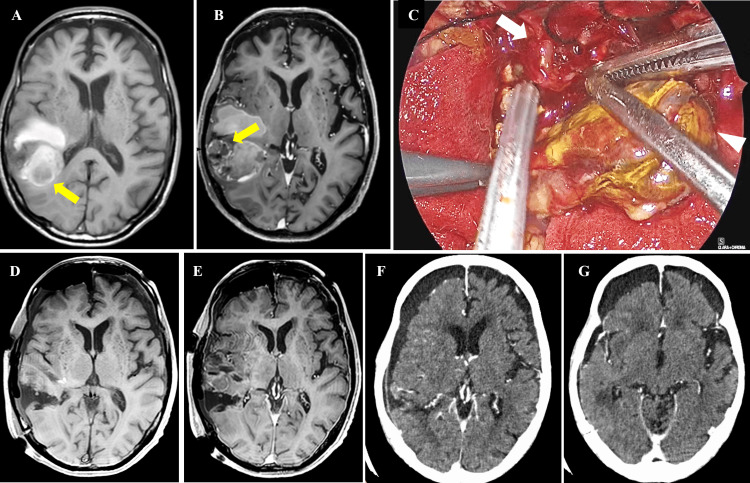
Radiological images during the disease course and a representative intraoperative photograph A, B: Shows MRI taken approximately 2.5 months after the onset of brain metastasis. A: New hemorrhage in the right temporal lobe on T1WI (arrow). B: A new enhancement corresponding to the previously noted elevated perfusion area (arrow), confirming the presence of a tumor. C: Shows neuroendoscopic intraoperative findings. Arrow: tumor adjacent to the hematoma, arrowhead: hematoma component. D, E: Shows postoperative MRI. D: T1WI. E: Gd-T1WI. F, G: Shows contrast-enhanced CT images obtained 75 days after the pathological diagnosis of brain metastasis. MRI, magnetic resonance imaging; T1WI, T1-weighted image; CT, computed tomography.
